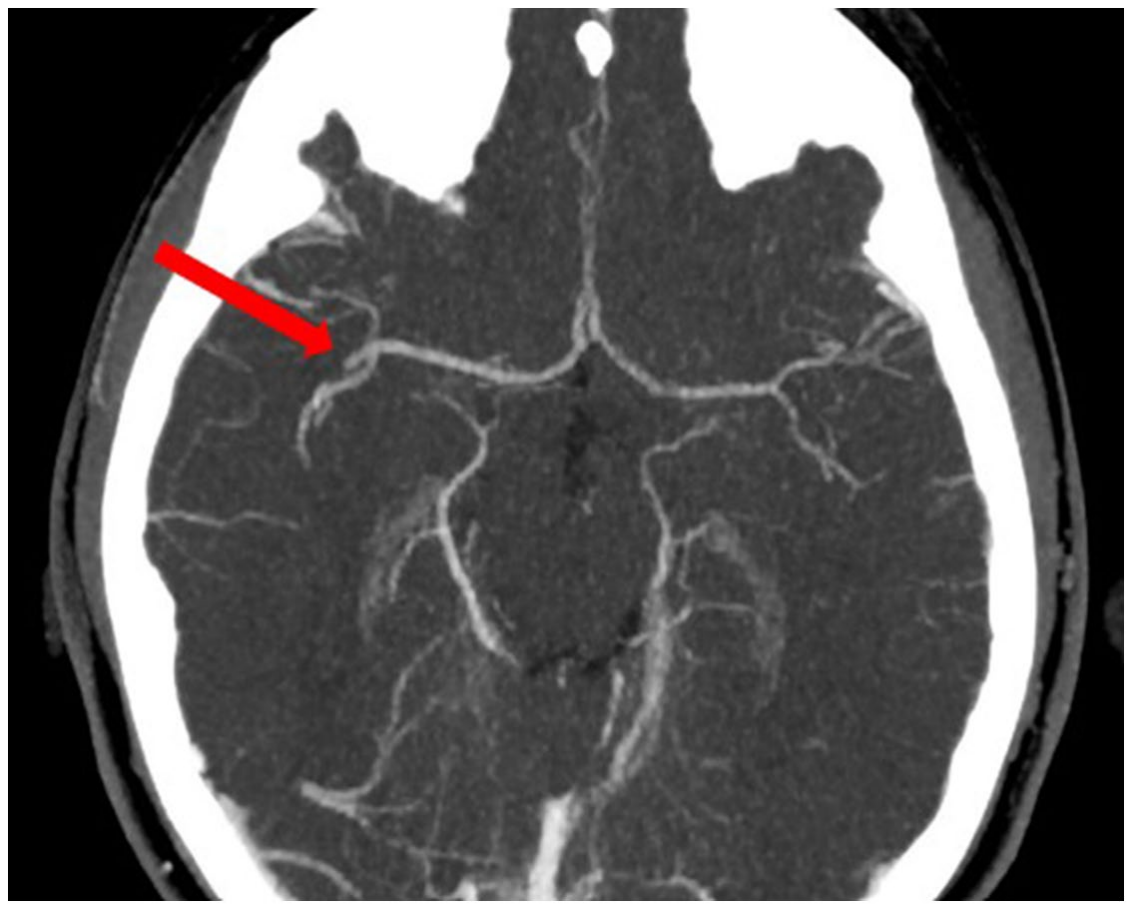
Angio-CT—occlusion of the M2 segment of the right middle cerebral artery before thrombectomy.
